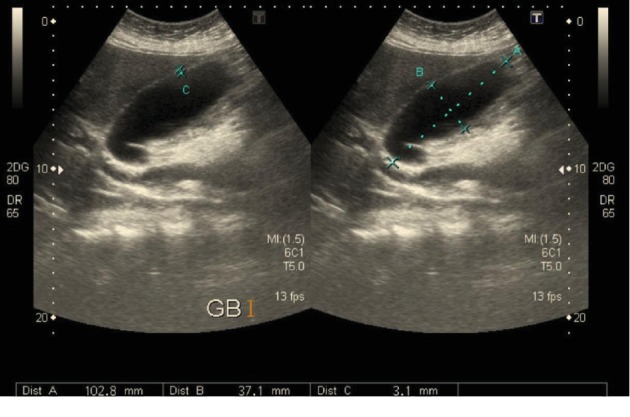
Transabdominal ultrasonography showing a distended gall bladder (102.8 × 37.1 mm) with a thin wall (3.1 mm) and tortuous dilated cystic duct with no intraluminal gallstones or pericholecystic fluid.
